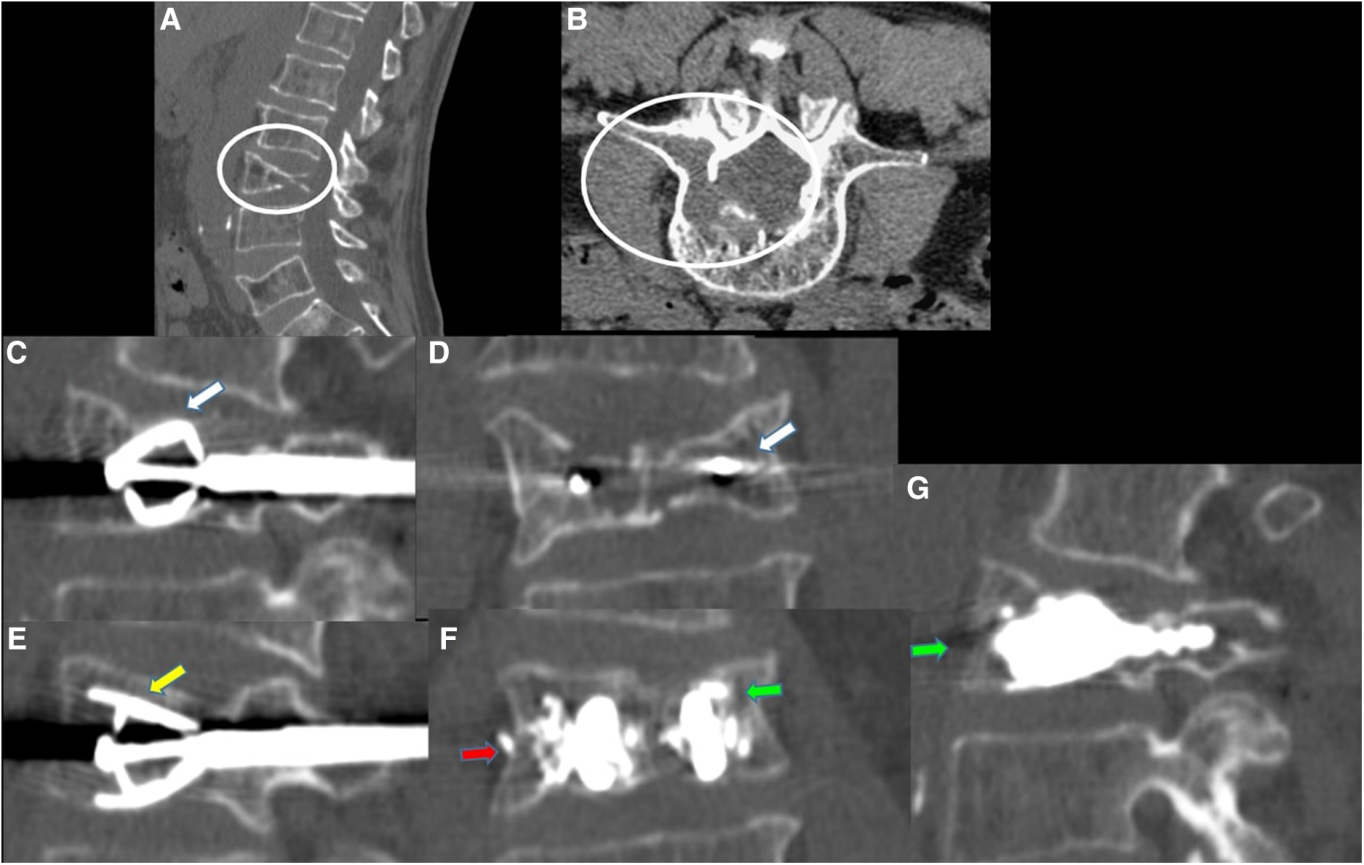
62-year-old male with a diagnosed MM with lumbar pain. A-B: Sagittal (A) and axial (B) CT scan showing L3 vertebra compression fracture, with significant reduction in the height of the posterior wall, with lytic lesion which also extends to the right pedicle [white circle in (A,B)]. (C–E): CT MPR (Multiplanar) in sagittal (C–E) and coronal (D) planes images; after preparation of both sides, two SpineJack® (white arrows), were inserted into the vertebral body and were gradually and simultaneously deployed [yellow arrow in (E)]. (F–G): CT MPR in coronal (F) and sagittal (G)plane images; Post procedure control images showed a correct expansion of the vertebra with a homogeneous distribution of the vertebral cementum. Minimum right lateral cement leak was observed [red arrow in (F)].
